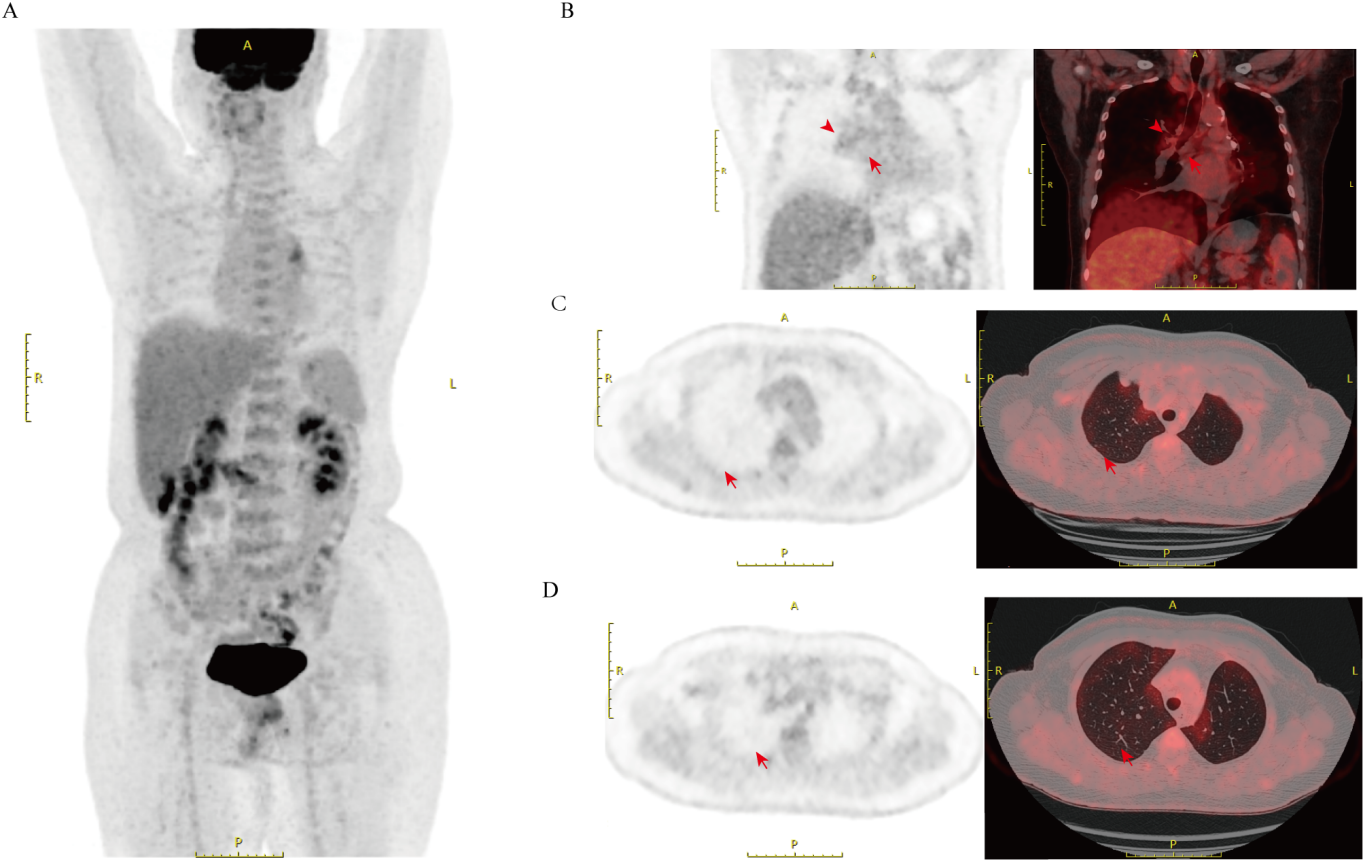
PET/CT imaging of the patient after steroid treatment (A) Follow-up PET/CT imaging of the same patient after treatment. (B) The mediastinal and hilar lymph nodes previously showing hypermetabolism are now normalized, with no high signal observed. (C) The previously observed hypermetabolic lesion along the pleural surface has resolved, with no visible high signal (arrows). (D) The hypermetabolic lesions in the right upper lobe have disappeared, with SUVmax no longer elevated (arrows).
